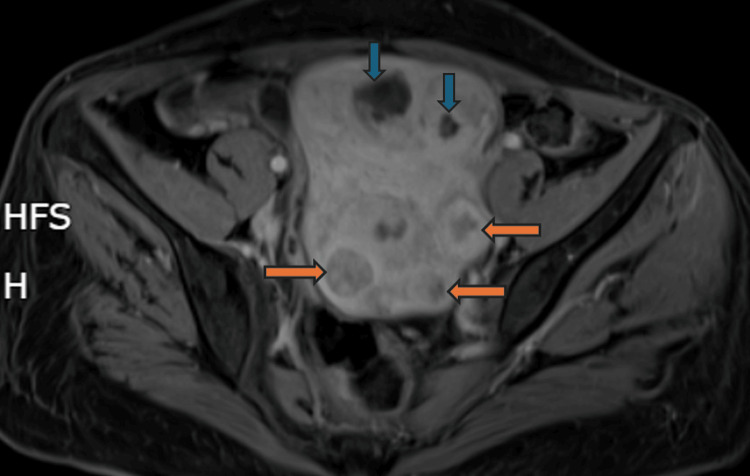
Pelvic MRI Pelvic MRI showing a polymyomatous uterus with myomas classified as International Federation of Gynecology and Obstetrics (FIGO) 4, 5 (orange arrows), with some showing cystic degeneration (blue arrows).
